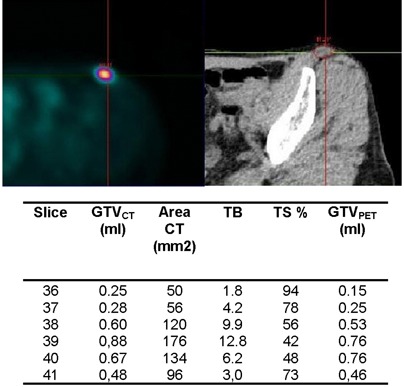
Example showing a good agreement between ${\text{GTV}}_{\text{PET}}$ (2.9 ml) and ${\text{GTV}}_{\text{CT}}$ (3.2 ml) in a medium sized lymph node with variable cross‐sectional dimensions, showing a highly variable TB ratio in different slices. Also shown is derivation of the ${\text{GTV}}_{\text{PET}}$.
